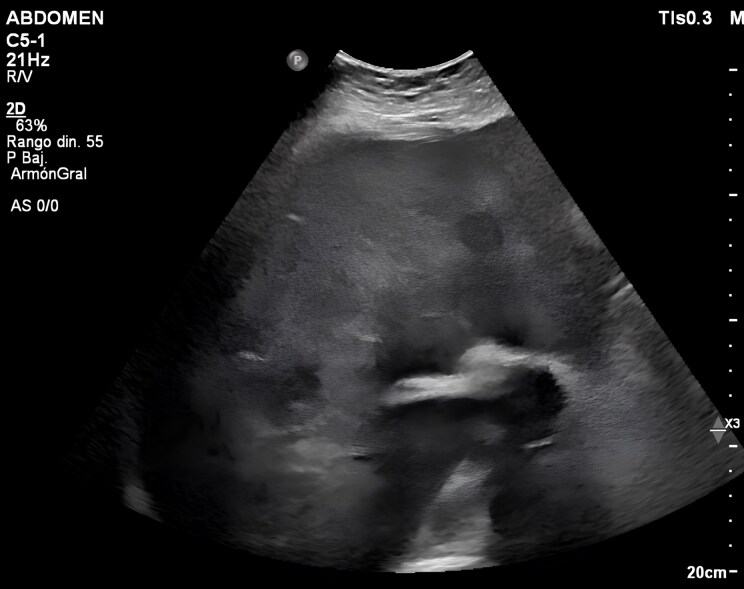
Abdominal ultrasound showing signs of chronic liver disease with multiple hypoechoic hepatic lesions.
